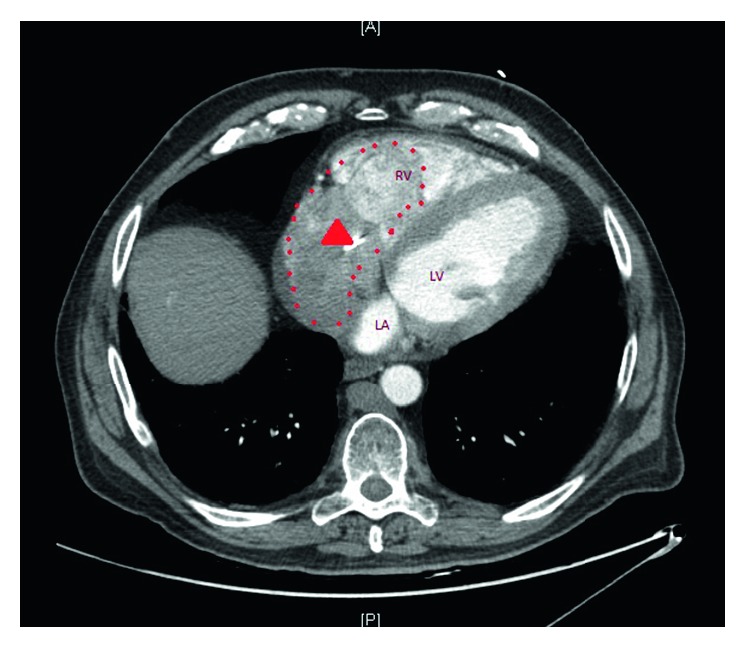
CT angiography of thorax displaying a suspected mass (dotted line) in the right side of the heart embedding the defibrillator wire (in red arrow head).
